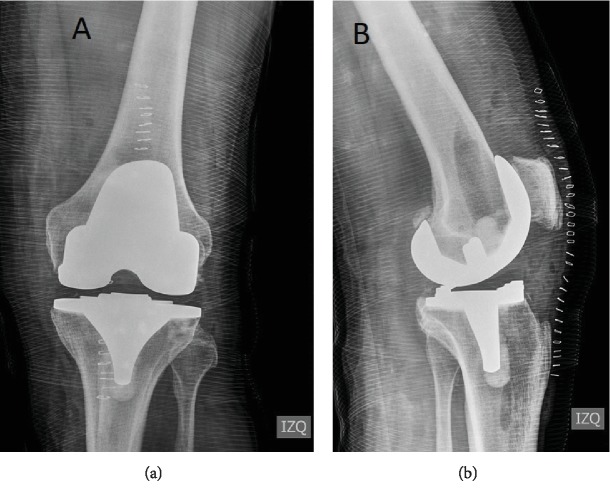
Immediately post knee arthroplasty radiograph: (a) anteroposterior left knee view and (b) lateral left knee view.
